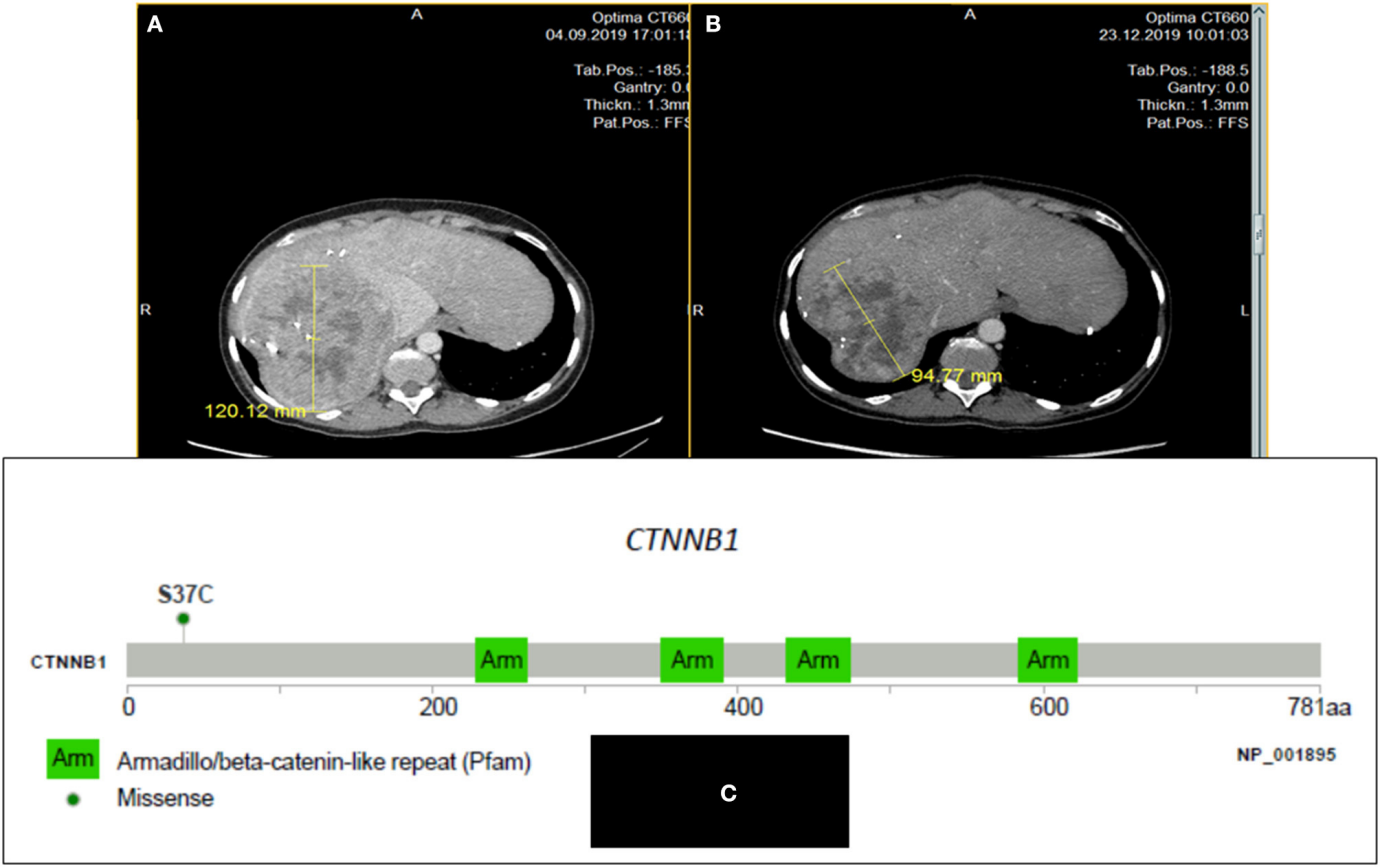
CT scan images of the large liver metastasis of a pancreatoblastoma (patient # 1) in a 33 year-old woman: before (A) and after six cycles (B) of FOLFIRINOX regimen: minor response; (C): Lolliplot of CTNNB1 gene showing the S37C mutation in exon 3.
